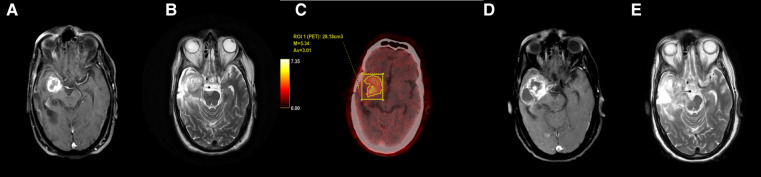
Axial T1 + Gd (A) and T2-weighted (B) images in a case of glioblastoma shows T2 intermediate signal intensity areas with thick rim enhancement. FET-PET (C) shows increased uptake (T/W ratio: 4.85) in the same area. Follow-up MRI (D,E) after 3 months showed increase in size of both solid and cystic components with likely infiltration into the cerebral peduncle. These features confirmed tumor recurrence.
